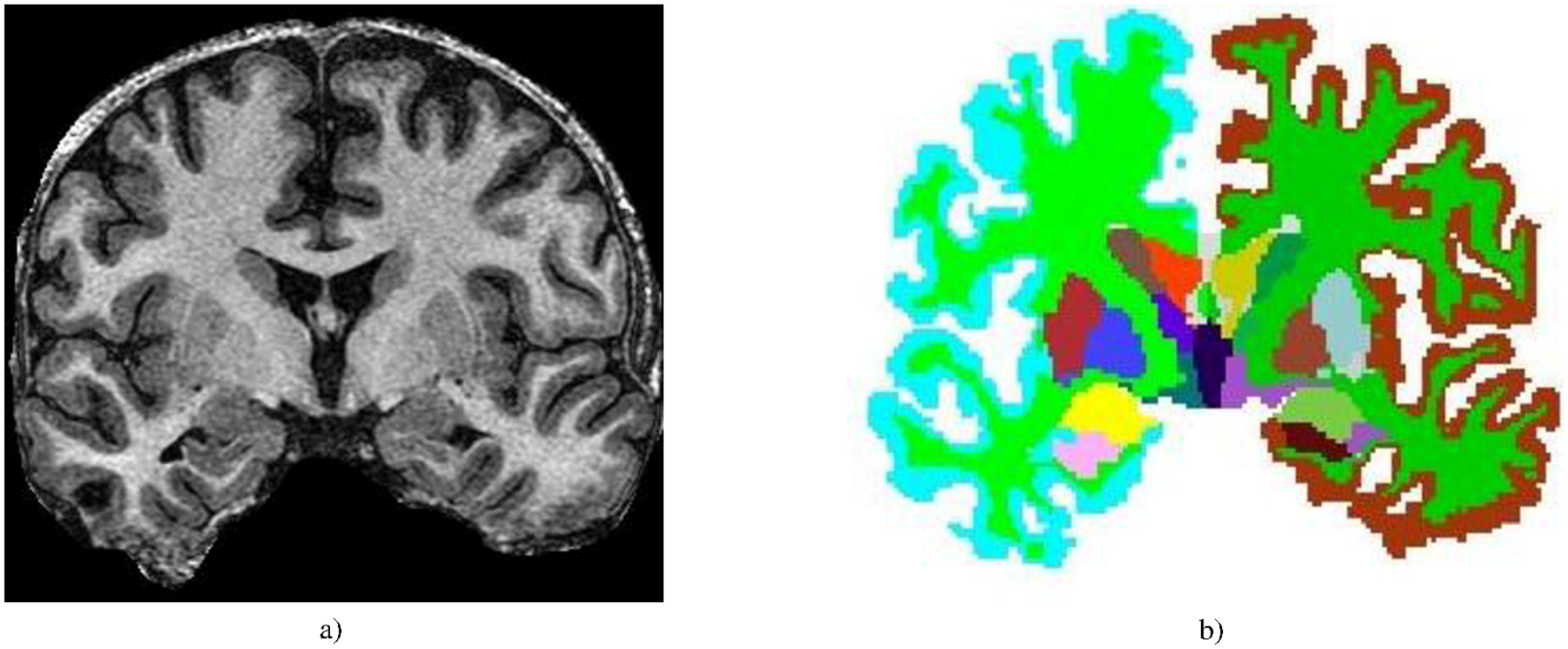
a) A coronal T1-weighted brain MRI. b) Brain structures segmented by FreeSurfer.
